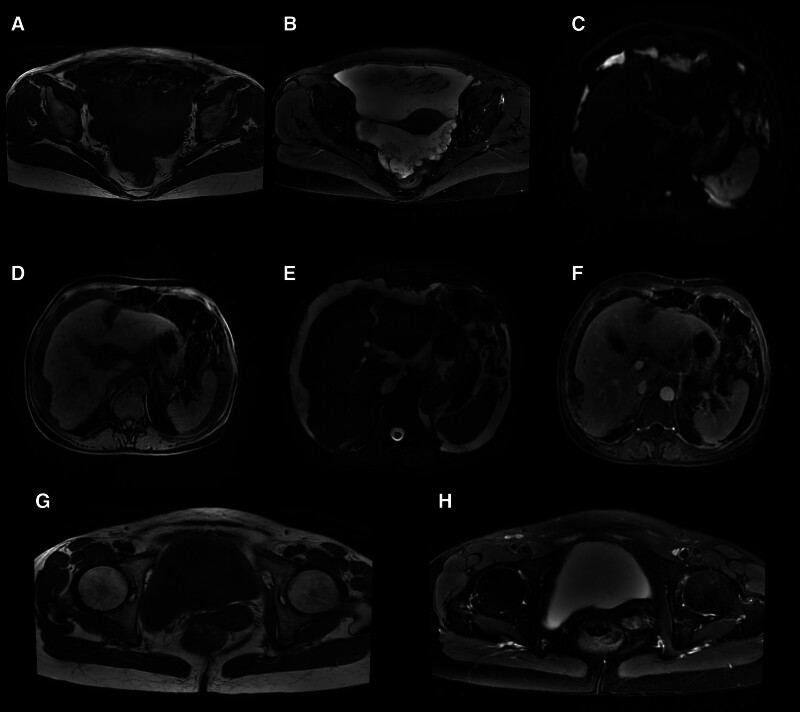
Representative imaging findings. (A) Ascites shows low signal intensity on T1WI. (B) Ascites shows high signal intensity on T2WI. (C) Irregular liver margins and perihepatic abnormal signals with high signal intensity on DWI. (D) Irregular liver margins and perihepatic abnormal signals with low signal intensity on T1WI. (E) Irregular liver margins and abnormal perihepatic signals with high signal intensity on T2WI. (F) Perihepatic abnormal signals with delayed-phase enhancement. (G) Abnormal cervical signal with slightly high intensity on T1WI. (H) Abnormal cervical signal with high intensity on T2WI. DWI = diffusion-weighted imaging, T1WI = T1-weighted imaging, T2WI = T2-weighted imaging.
